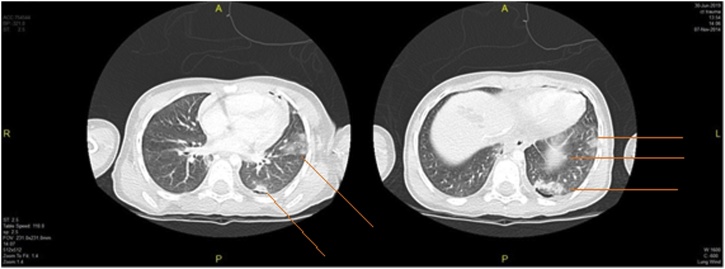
CT trauma revealed left lung contusions (orange lines).
